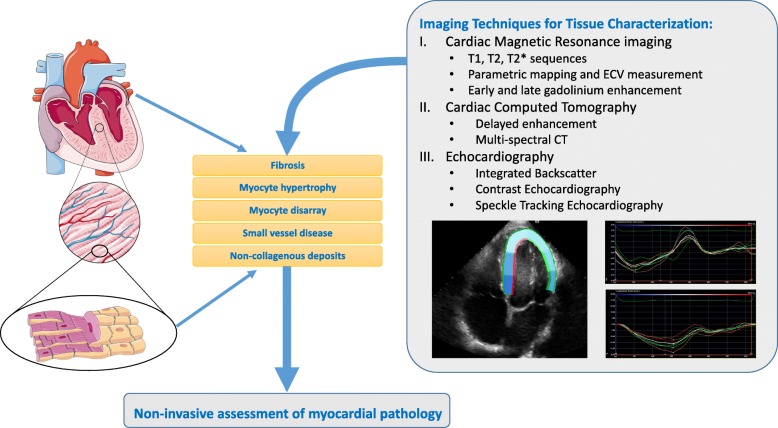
Overview of imaging techniques for tissue characterisation. Accurate characterisation of important myocardial features, such as fibrosis or myocyte hypertrophy, would allow non-invasive diagnosis of myocardial pathology, potentially allowing the development of personalized therapies. (Image contains material licensed under CC-BY 3.0 from https://smart.servier.com/). Abbreviations: CT, computed tomography; ECV, extracellular volume
